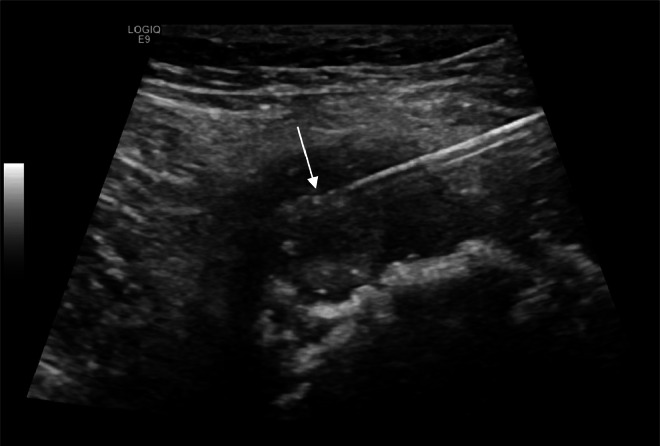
Ultrasound-guided biopsy of the proximal left tibia mass. Grayscale image shows the needle tip (arrow) in the peripheral noncalcified component of the mass. BioPince^TM^ core biopsy device was used with a 16 gauge needle and 23 millimeter throw.
